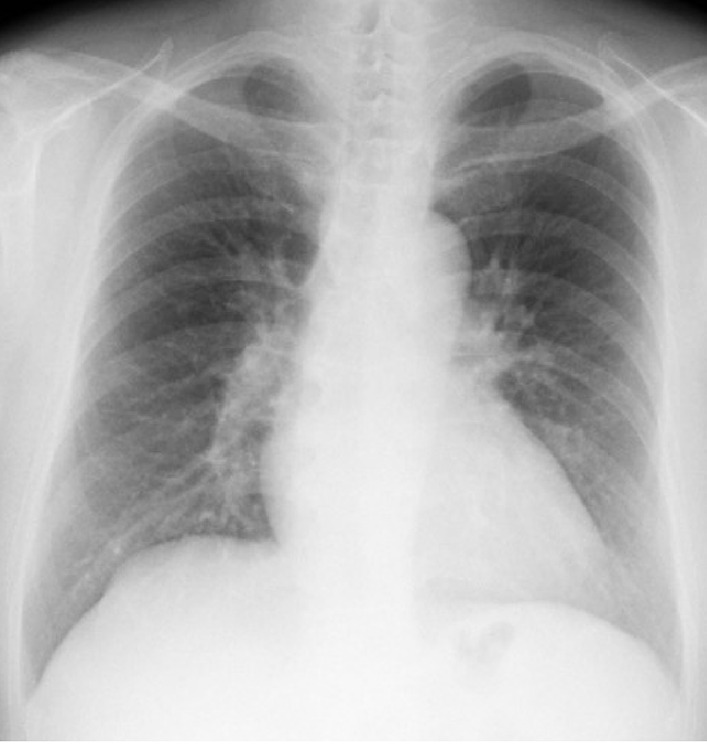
Chest radiograph on admission. The radiograph showed no significant findings
